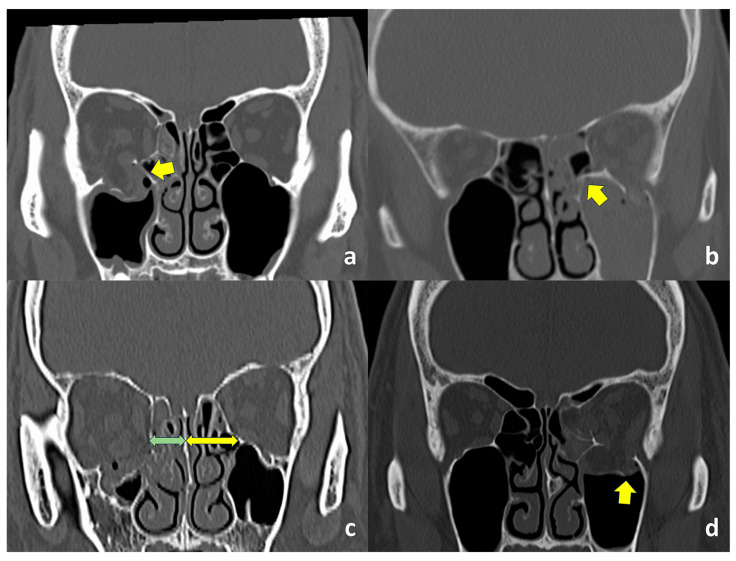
Computed tomographic (CT) findings. (a). The inferomedial orbital strut is not fractured (arrow). (b). The inferomedial orbital strut is fractured (arrow). (c). The distance from the junction between the orbital floor and medial orbital wall and nasal septum is shorter on the affected side (green arrow), compared to the unaffected side (yellow arrow). (d). The orbital floor lateral to the infraorbital groove (arrow) is fractured.
